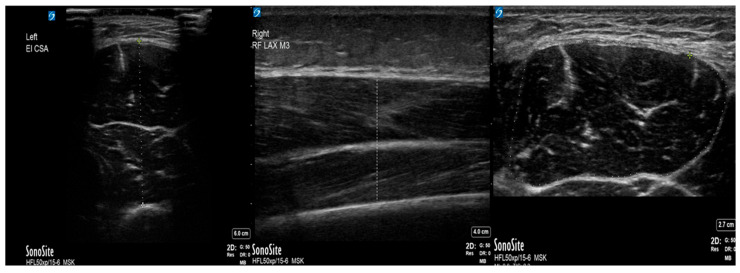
Representative ultrasound images of the quadriceps femoris muscle of a critically ill pediatric patient. Measures of the short axis (left panel), long axes (middle panel) and cross-sectional area (right panel) of the muscle were assessed.
